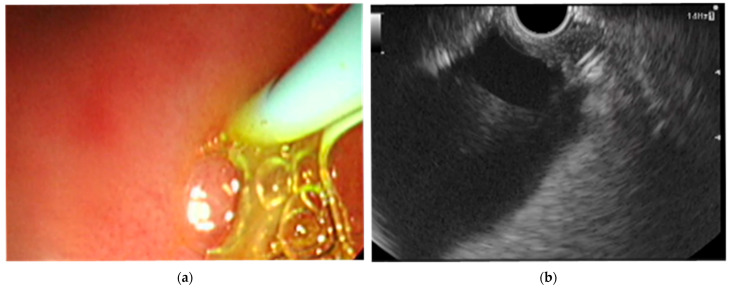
ENBD tube was inserted into the abdominal cavity. (a) Endoscopic view. (b) Endoscopic ultrasound (EUS) view.
